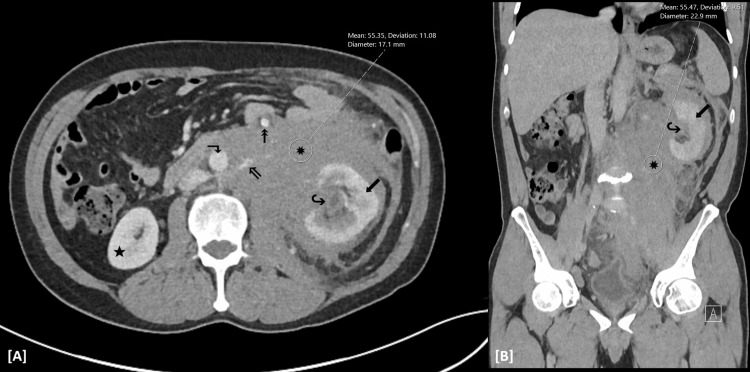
Contrast-enhanced CT of the abdomen in axial (A) and coronal (B) planes at the level of the L3 vertebral body. A large infiltrative soft-tissue density lesion (~55 HU) is present within the left retroperitoneum (✸), forming a rind-like soft-tissue mantle around the left kidney. The lesion encases the left renal vasculature, including the left renal vein (⇖) and renal hilum (↪), with associated left kidney pelvocaliectasis. There is also regional mass effect, with rightward and anterior displacement of the abdominal aorta (↴) and inferior vena cava (IVC) and anterior displacement of the inferior mesenteric vein (↟). Note the diffuse swelling/enlargement of the left renal cortex and delayed nephrogram (⬋) compared with that of the right kidney (★).
